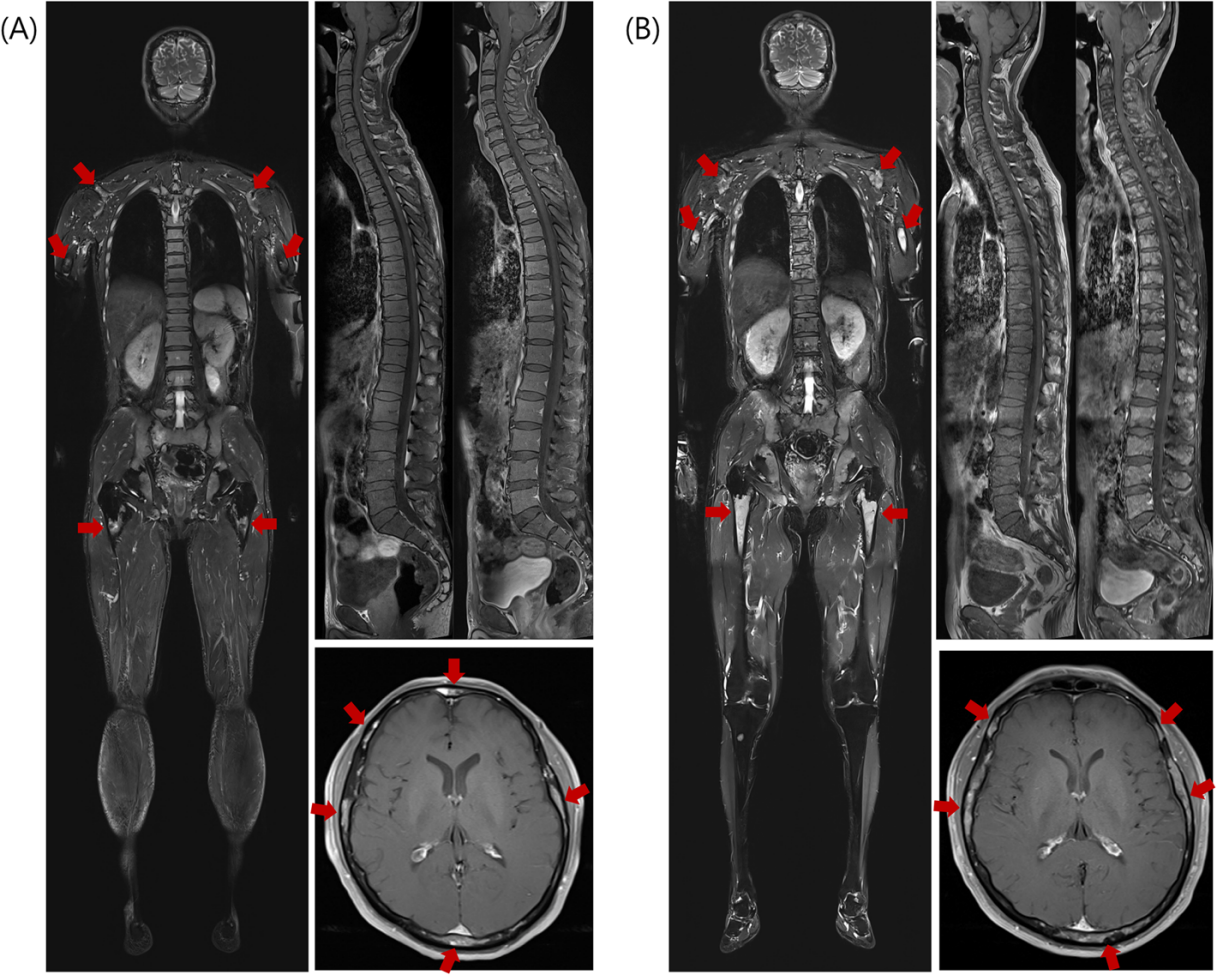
A 56-year-old male with multiple myeloma showing disease progression. Coronal T2WI (left) taken before chemotherapy (a) shows diffuse high signal intensity in the bone marrow (arrows). Sagittal CE T1WI (upper right) shows diffuse enhancement of bone marrow in the whole spine. Coronal enhanced T1WI (lower right) shows multifocal bone marrow enhancement in the calvarium. MRI at 14 months after initiation of chemotherapy and ASCT (b) shows a further increase in the signal intensity and extent of diffuse bone marrow lesions (arrows) on coronal T2WI (left) and sagittal T1WI (upper right). Axial T1WI (lower right) shows enlarged focal lesions in the calvarium, indicative of disease progression
